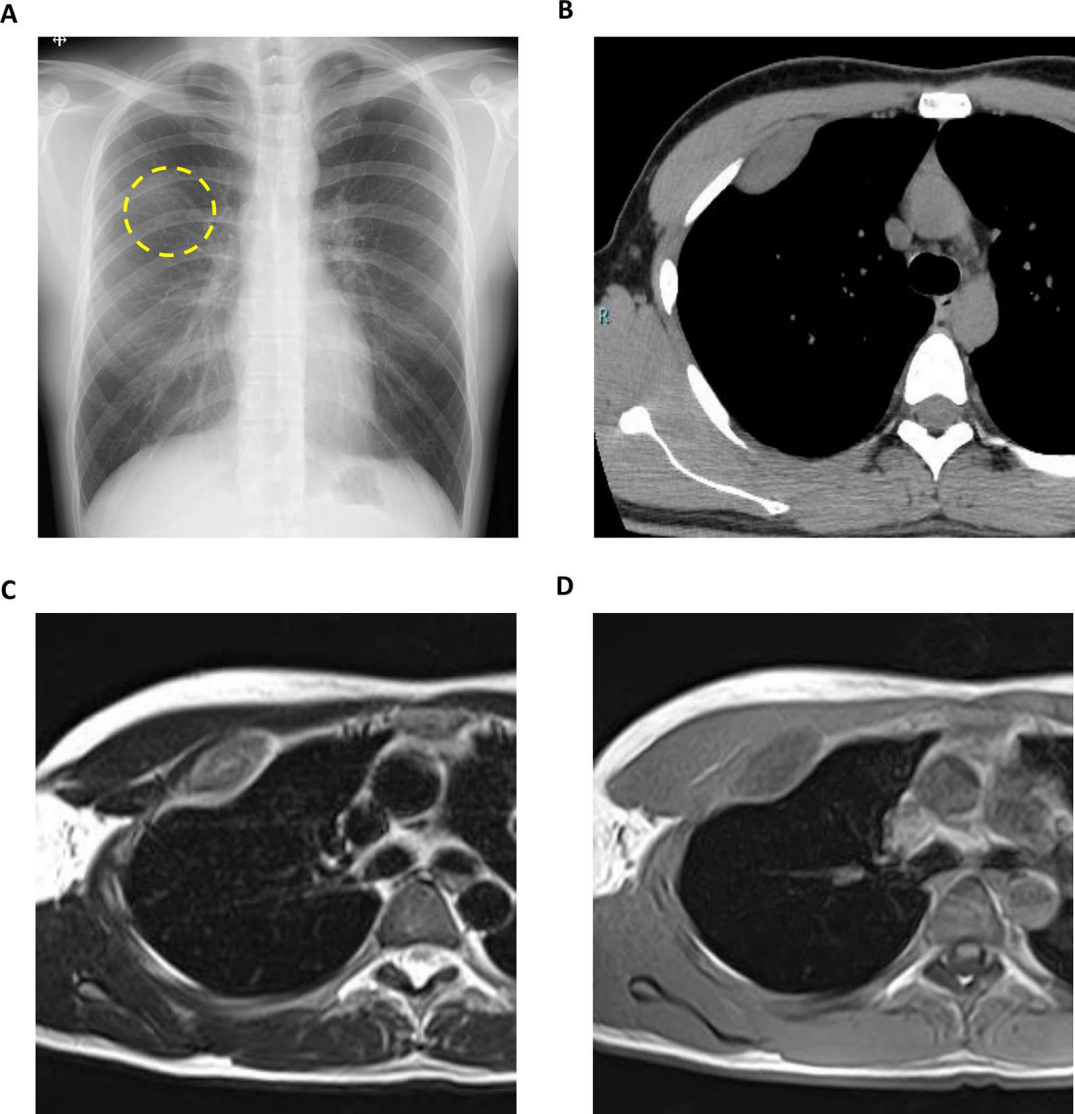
a Chest radiography showed an abnormal shadow in the right upper lung field (dotted circle). b Chest computed tomography showed a 30-mm mass in the right second intercostal space. c T2-weighted MRI scan showed a high-intensity area in the right second intercostal space without evidence of peritumoral invasion. d T1-weighted MRI scan showed intermediate intensity area in the right second intercostal space. MRI: Magnetic resonance imaging
